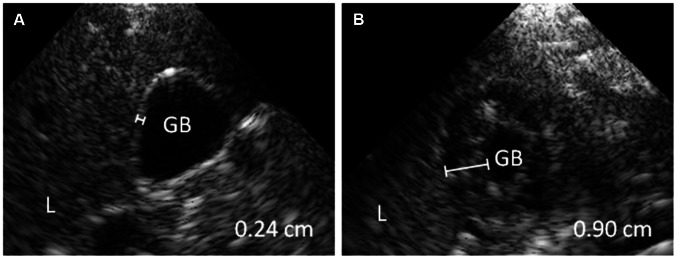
Ultrasonography findings showing a normal and thickened gallbladder wall in dengue patients. Handheld ultrasonography performed at the bedside with the patient in supine position during examination. (A) normal gallbladder wall (<0.30 cm) (B) and thickened gallbladder wall. The gall bladder wall is thickened with 0.90 cm and has a subserosal fluid layer, giving the gallbladder wall a multiple layer aspect. Abbreviations: L: liver; GB: gallbladder lumen.
